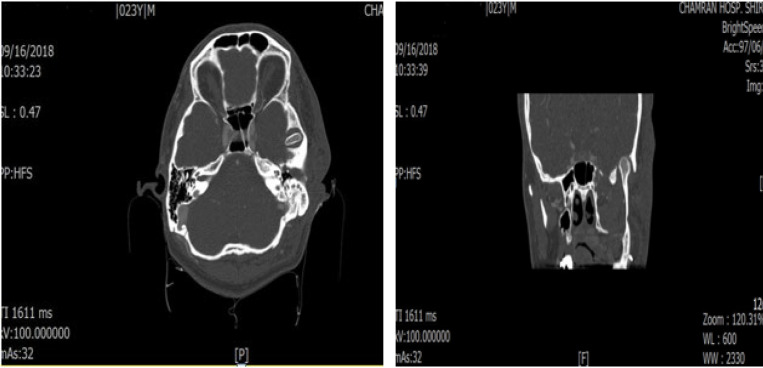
Axial and coronal view of computed tomography images show intrusion of mandibular condyle into middle cranial fossa
